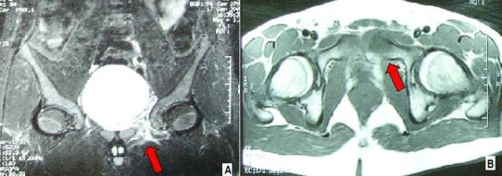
(A, B) Pelvic/hip MRI demonstrating slightly increased signal in the left pubis, adjacent to the pubic symphysis, suggesting ostietis pubis or osteomyelitis. Increased signal reveals mainly to the proximal segment of the obturator internus muscle and hereupon to the obturator externus muscle with little fluid collection, and slight edema of the soft tissue, but with no abscess cavities.
